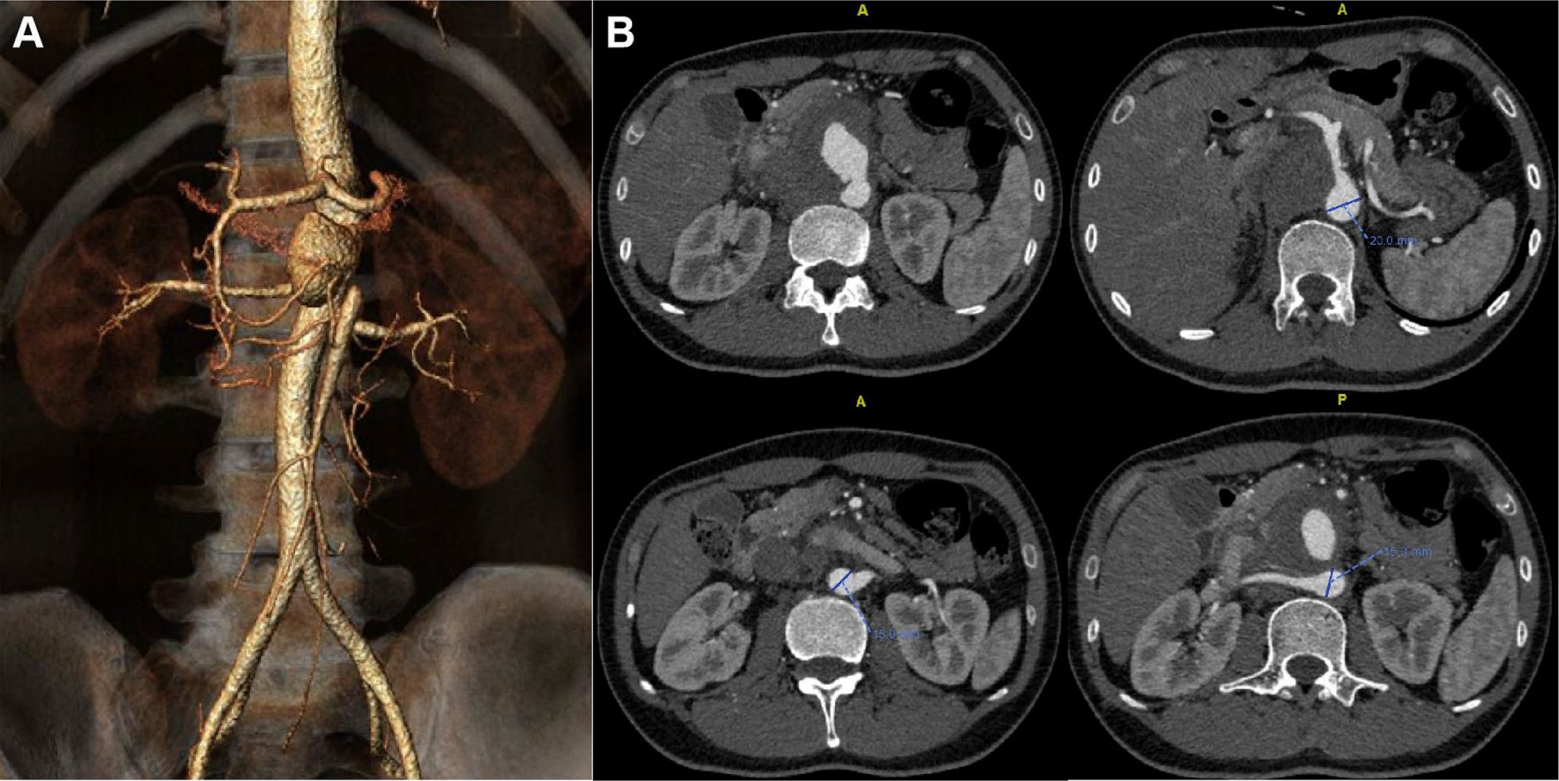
(A) Preoperative computed tomography angiography (CTA) with volume rendering reconstructions demonstrated paravisceral aortic pseudoaneurym. (B) Rupture location of pseudoaneurysm and aorta dimension at the level of visceral arteries
